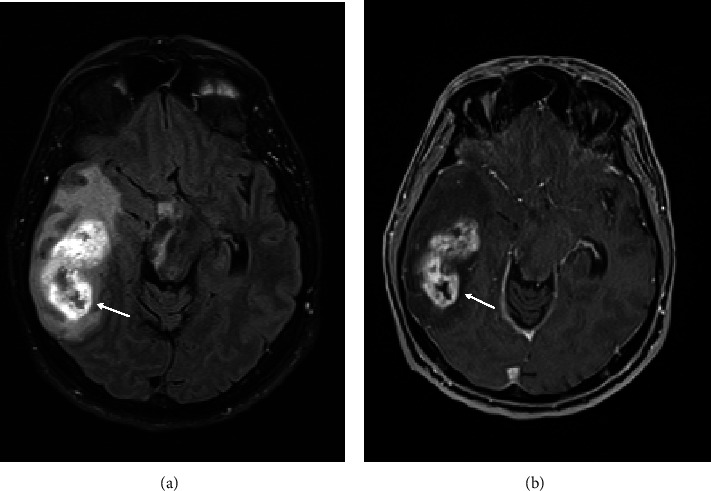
Axial, contrast-enhanced MR images at the middle cranial fossa level. (a) FLAIR and (b) T1-weighted images demonstrate an irregular enhancing mass lesion with central areas of necrosis within the right temporal lobe.
